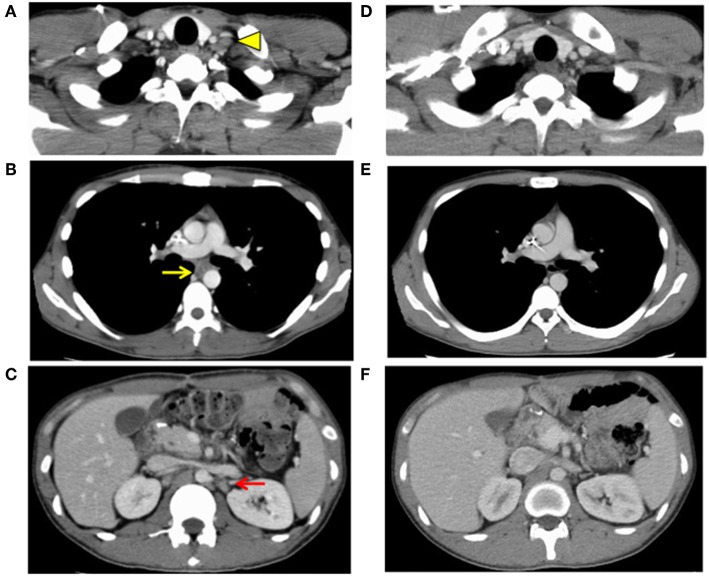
Images of computed tomography 1 month after surgery (A–C) and after finish of the nivolumab threatment (D–F). Lymph node metastasis was observed (A) in the subclavicle (#104L, yellow arrowhead), (B) mediastinum (#108L, yellow arrow), and (C) paraaorta (#16b1, red arrow), indicating distally metastatic recurrence of the carcinoma (progressive disease). Lymph node metastasis of (D) the subclavicle (#104L), (E) mediastinum (#108L), and (F) paraaorta (#16b1) had vanished, indicating a complete response.
